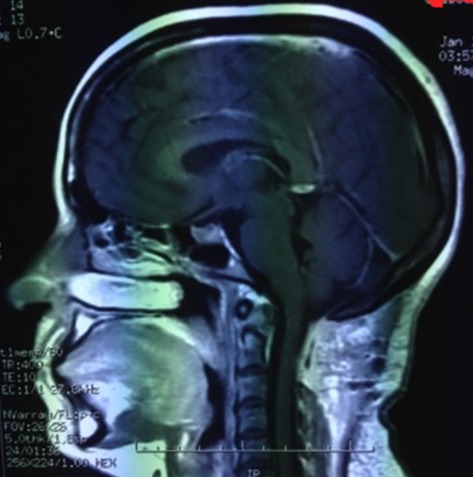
Postoperative T1 Weighted sagittal MRI shows total removal of the lesion
